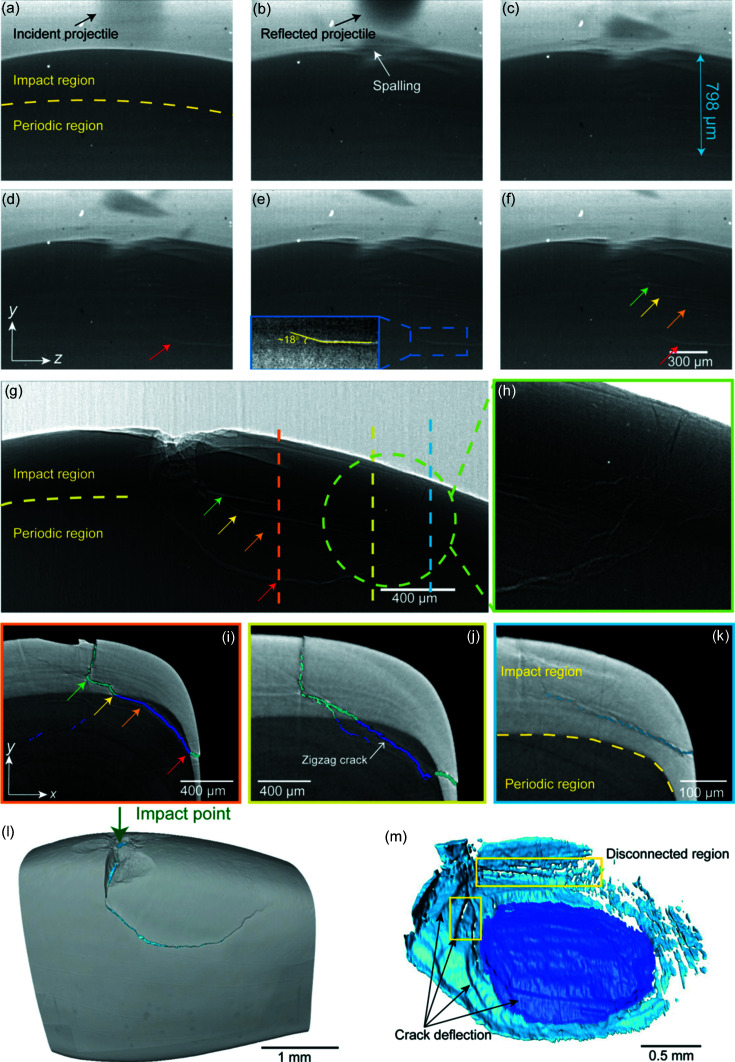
Dynamic structure deformation and crack evolution observed via *in situ* 2D fast imaging and high-resolution 3D post mortem CT examination. (*a*)–(*f*) Snapshots of dactyl club under dynamic impact with time intervals of 6 µs. Catastrophic spalling and clear crack deflections can be spotted *in situ*. Image (*e*) shows the propagating crack starting to deflect towards (highlighted with the yellow line) the impacting point at 27 µs after the exact moment of impact, which grew further [indicated by the red arrow in (*g*)] and merged with another crack system nucleated right under the impact point as shown in (*g*). Image (*g*) displays a projection of the impacted dactyl club from an *ex situ* CT test at a similar perspective as in the *in situ* 2D imaging, with some major deflection sites indicated with arrows. Image (*h*) shows crack-branching behaviors while they were deflected towards the surface of dactyl club. (*i*)–(*k*) Slices of CT reconstruction of damaged dactyl club denoted in (*g*). The main crack was deflected around the interface of the impact-periodic region. Within the periodic region, the crack was deflected periodically forming a zigzag-shaped crack path. At the end of main crack, the crack branched into microcracks and propagated to the surface of dactyl club as shown in (*k*). (*l*) 3D rendering of damaged dactyl club. (*m*) 3D distribution of the main crack system developed in the dry dactyl club sample. Dark blue: cracks within the periodic region. Light blue: cracks within the impact region.
